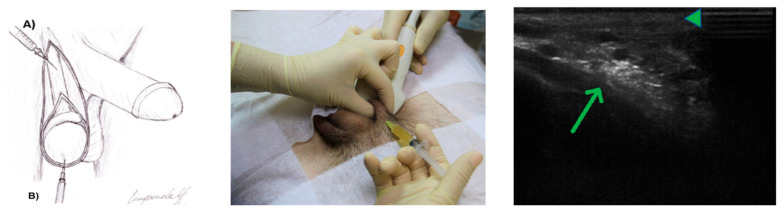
(Left)—Technique of PRP injections for male infertility: A—intracanalicular; B—intratesticular. (Center)—PRP injection technique for male infertility (intracanalicular under ultrasound control). (Right)—Movement of the PRP to the epididymis through 8 min (green triangle—epididymis; green arrow—intracanalicular deposition of PRP).
